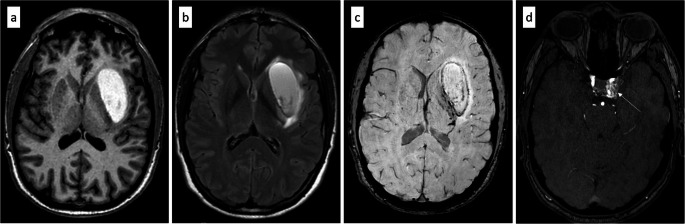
MRI demonstrates a lesion with imaging characteristics consistent with a late subacute hemorrhage in the region of the left external capsule, showing T1- and FLAIR-hyperintense internal signal (a, b), perifocal edema (b), and peripheral hemosiderin deposition on SWI (c). TOF imaging demonstrates arterialized flow signal within the left cavernous sinus (arrow in d)
